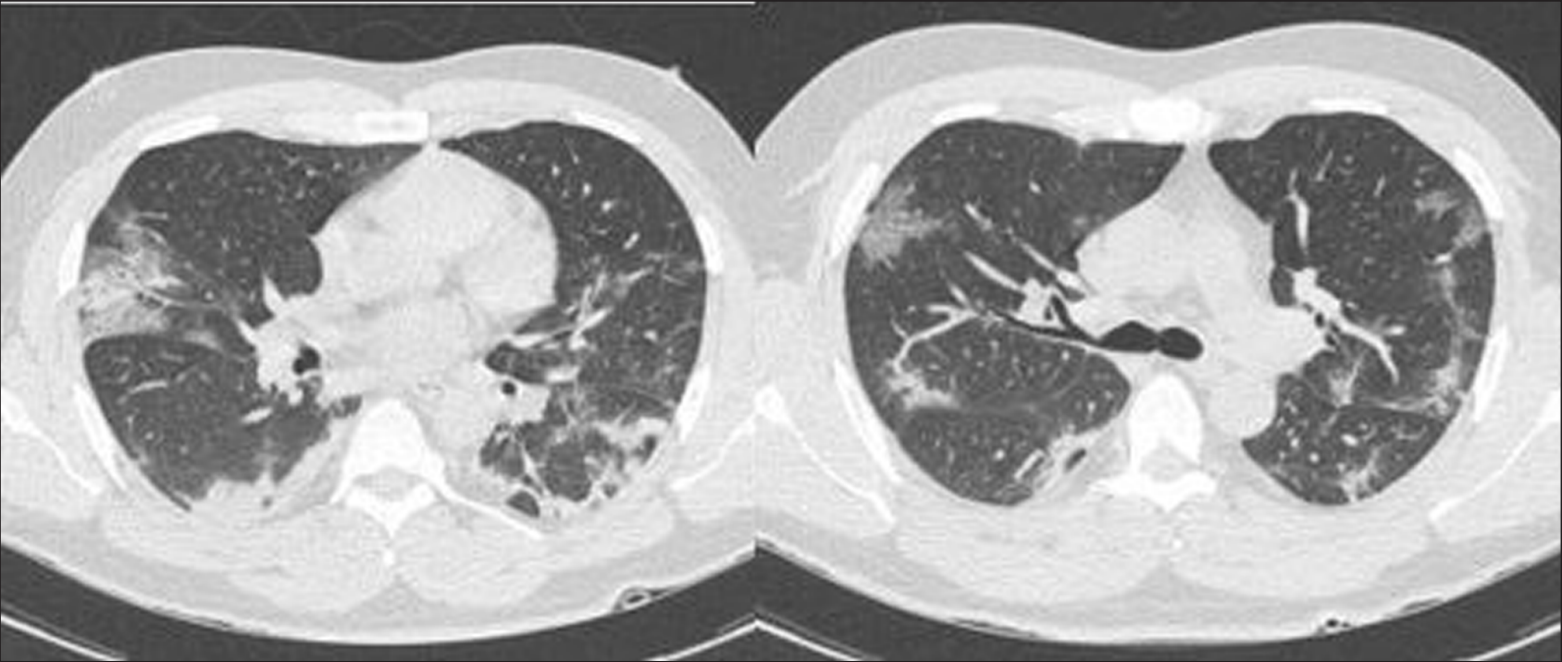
False-positive CO-RADS chest CT for COVID-19 when compared to RT-PCR test: 61-year-old woman with metastatic adenocarcinoma under chemotherapy. CO-RADS score of 4. No significant change on CT when comparing to previous scans (not available to the reader at the time of scoring).
